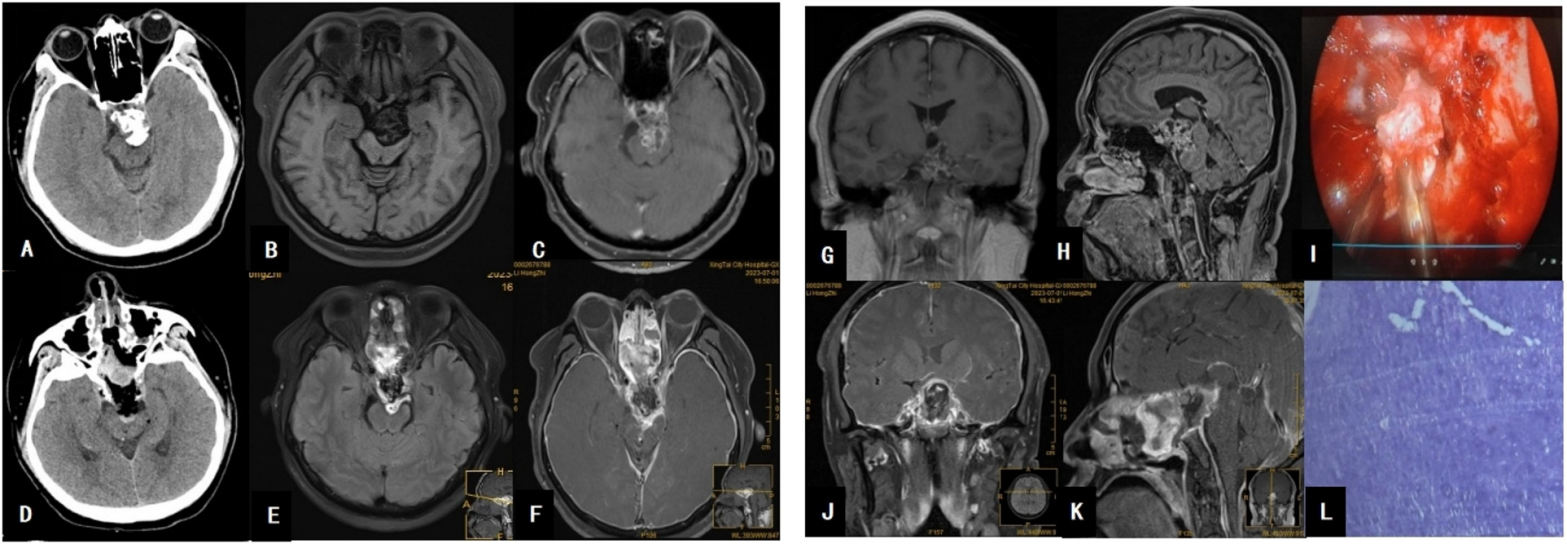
(A) Brain CT shows a hyperdense mass posterior to the sella turcica. (B) MRI T1-weighted image reveals a hypointense signal posterior to the sella turcica and anterior to the brainstem. (C) MRI T1 axial contrast-enhanced image demonstrates heterogeneous enhancement of the large tumor, which encases the basilar artery. (G) MRI T1 coronal contrast-enhanced image shows the tumor’s close relationship with the left cavernous sinus. (H) MRI T1 sagittal contrast-enhanced image reveals tumor compression on the brainstem, resulting in brainstem deformation. (I) Intraoperative view of tumor resection; the tumor exhibits a firm texture. (D) Postoperative CT changes after tumor resection. (E,F,J,K) Postoperative MRI changes, indicating complete tumor resection. (L) Pathological image of the tumor.
